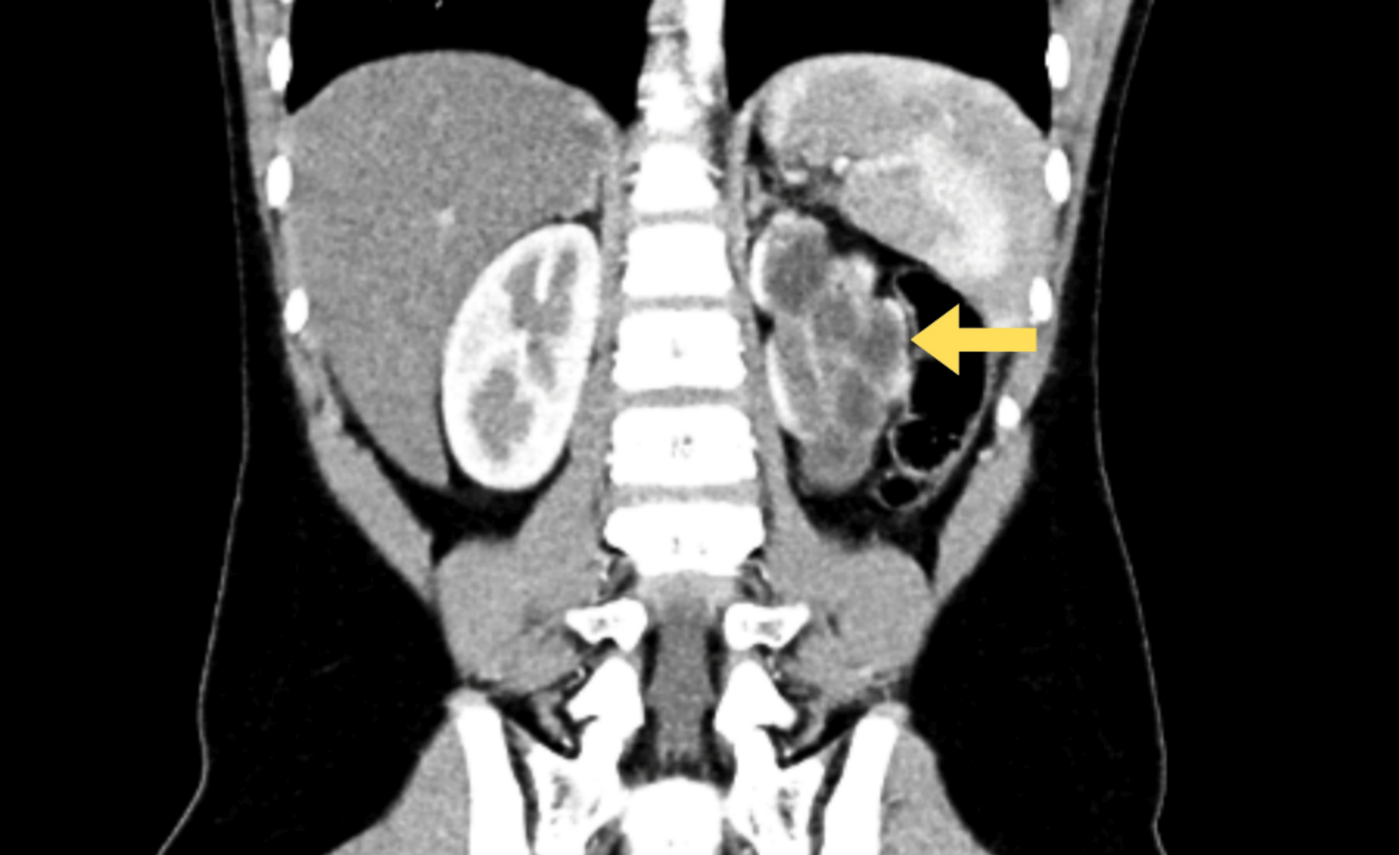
Reno-pelvic CT image revealing cortical thinning and calyceal ectasias of the left kidney.
